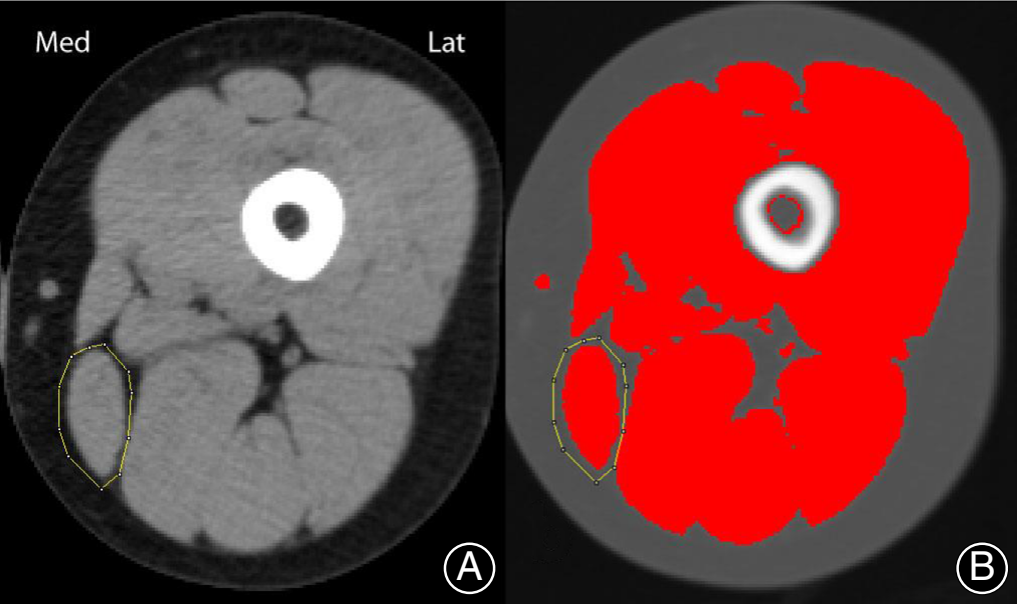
Computed tomography (CT) image of left thigh (A) and that treated with ImageJ (B)^78^.
